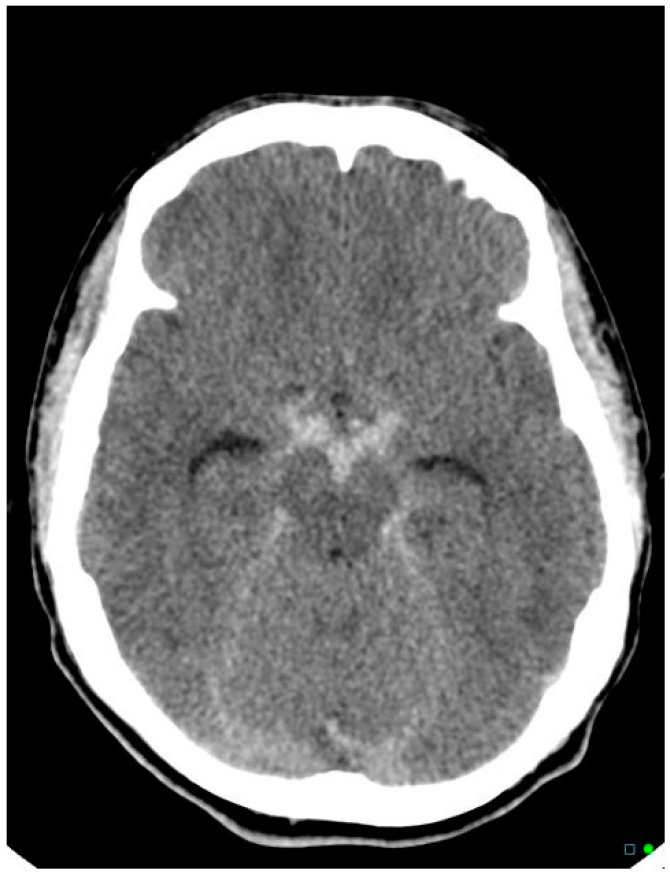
CT scan showed a thin layer of hyperdense subarachnoid blood encircling the upper brainstem, typical of a peri mesencephalic SAH. The 44-year-old patient presented with a GCS of 15 following a sudden-onset headache. CT angiography was normal.
